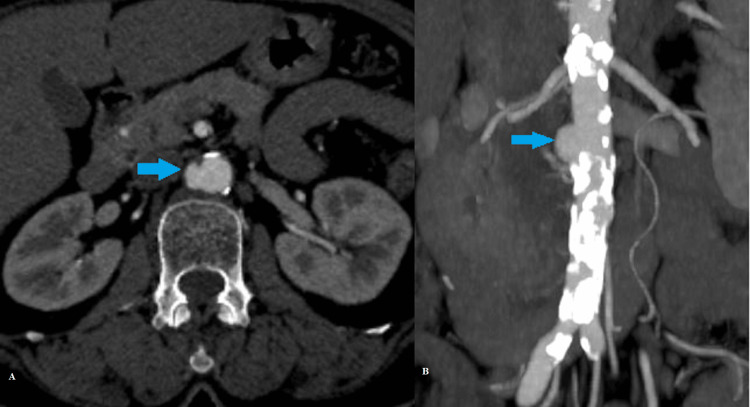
(A) Axial and (B) coronal CT angiography images show contrast-filled ulcer crater in the background of severe systemic atherosclerosis (blue arrows).
